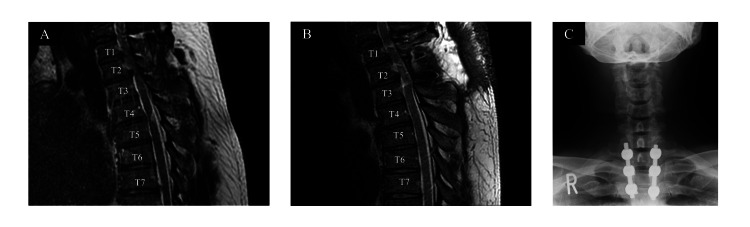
The images of MRI and X-ray for cervical and thoracic spine. A, MRI image of pre-operation; B, MRI image of post-operation; C, X-ray image of post-operation
